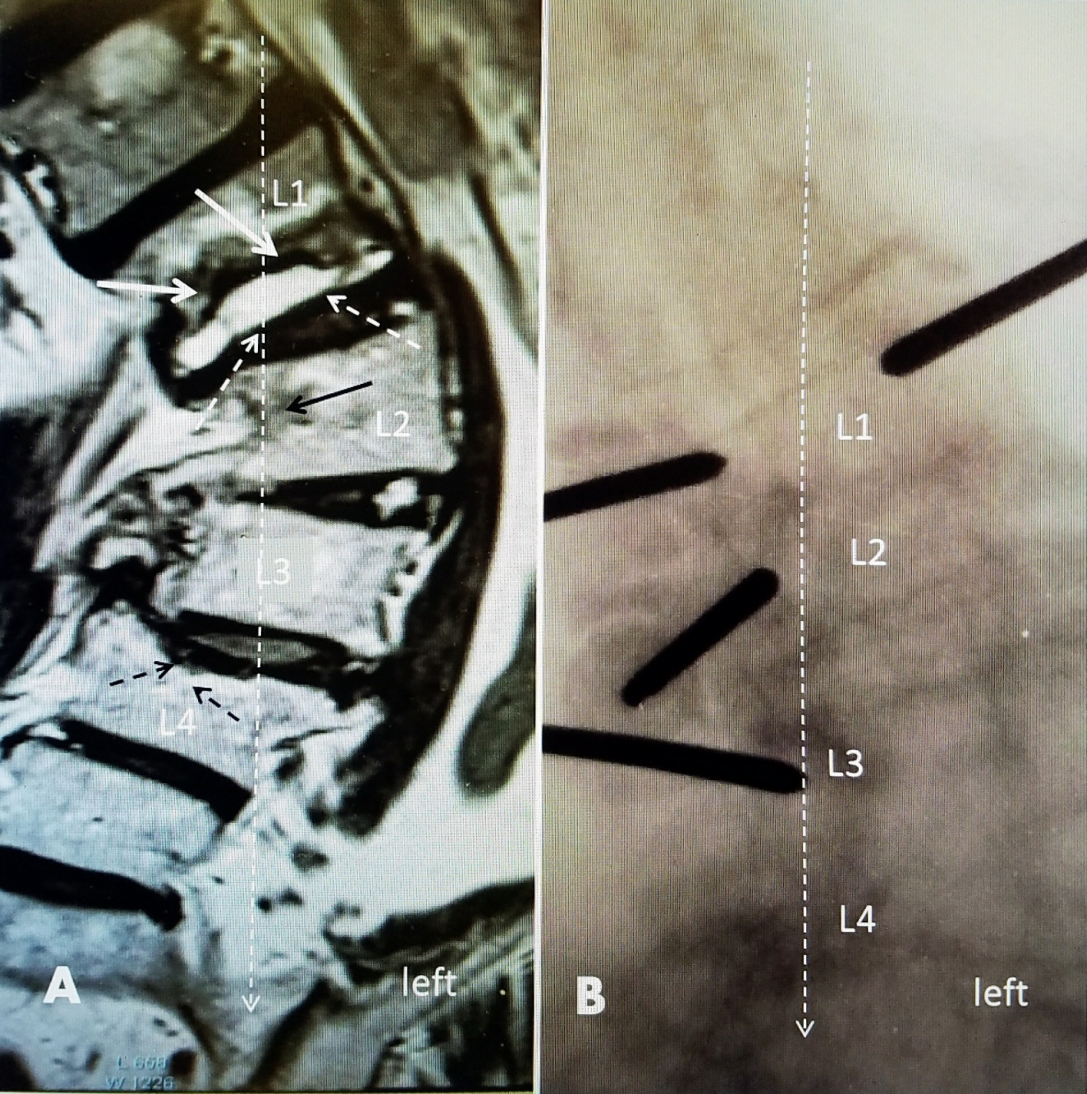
A 70-year-old female with pre-existing idiopathic scoliosis with a left convex curve of 50 degrees A: A sagittal magnetic resonance imaging (MRI) scan showing an L1 fracture with vertebral body edema of the inferior 50% of L1. There is an intervertebral fluid-filled T2 hyper-intense cleft inferiorly (white arrow). At L2 on the right, there is bone marrow edema (solid black arrow) under the L1 fracture. At L4, there is an old superior endplate fracture, without edema, and 20^o^ collapse to the center and right (dashed black arrows). B: Intraoperative image with the placement of vertebroplasty cannulas. There are two cannulas at the fractured L1. There is a single cannula in L2 and L3 in the concave part of the curve below the fracture to place cement for preventive vertebroplasty. Vertebral augmentation was performed bilaterally at L1 and unilateral cement injections at L2 and L3 were performed using Cortoss (Stryker, Kalamazoo, Michigan, USA) directly under the L1 fracture. The center line of the weight-bearing load (dashed, long white arrow) for the lower lumbar spine is seen passing through the center of L1 and the concave right side of both L2 and L3.
